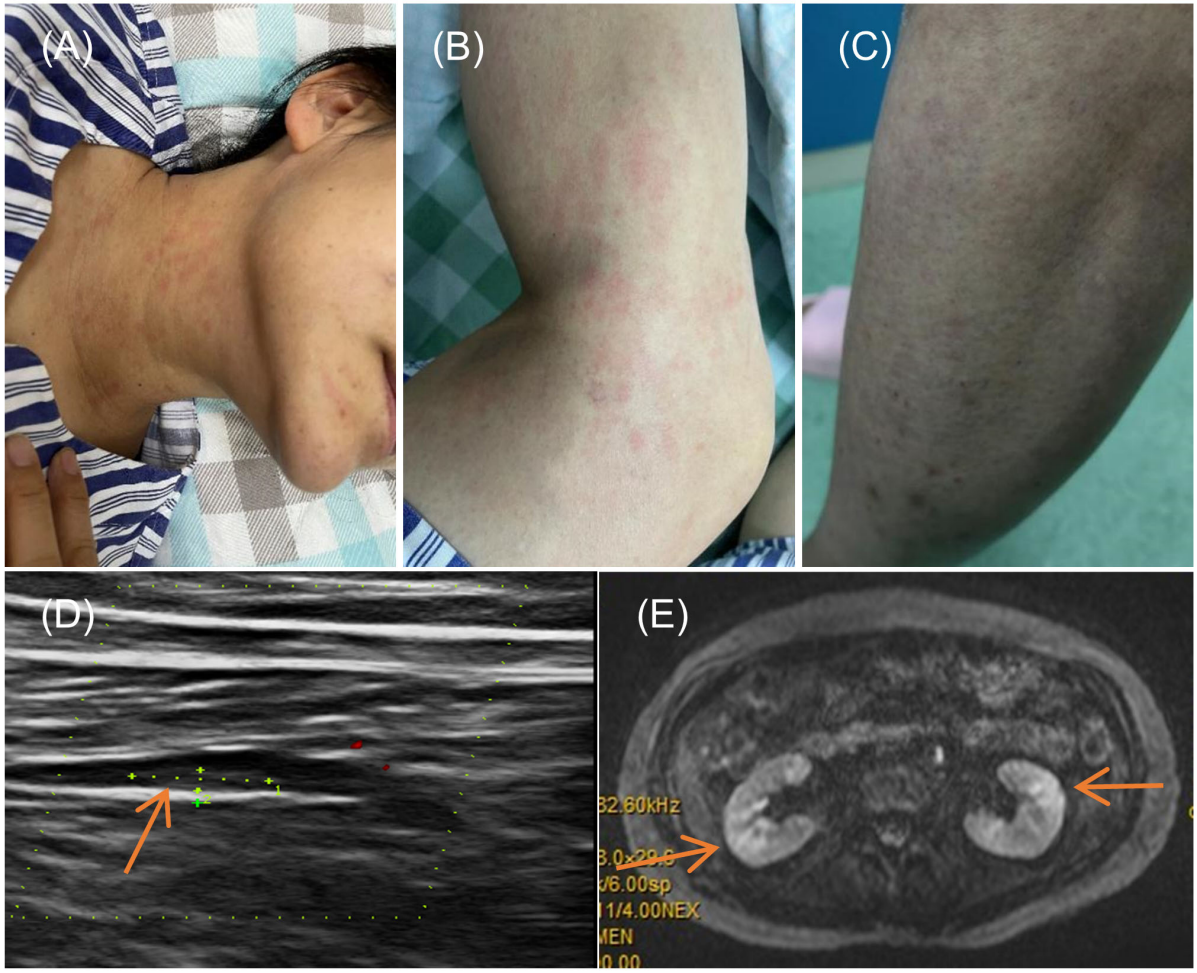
Symptoms and Imaging Results of the Patient. (A, B) Rash on the neck and arms of the patient; (C) Change in skin color of the lower limbs upon standing; (D) Doppler ultrasound of the lower limbs indicating local thrombus formation; (E) Diffusion-weighted imaging (DWI) sequence from an enhanced MRI of the kidneys.
